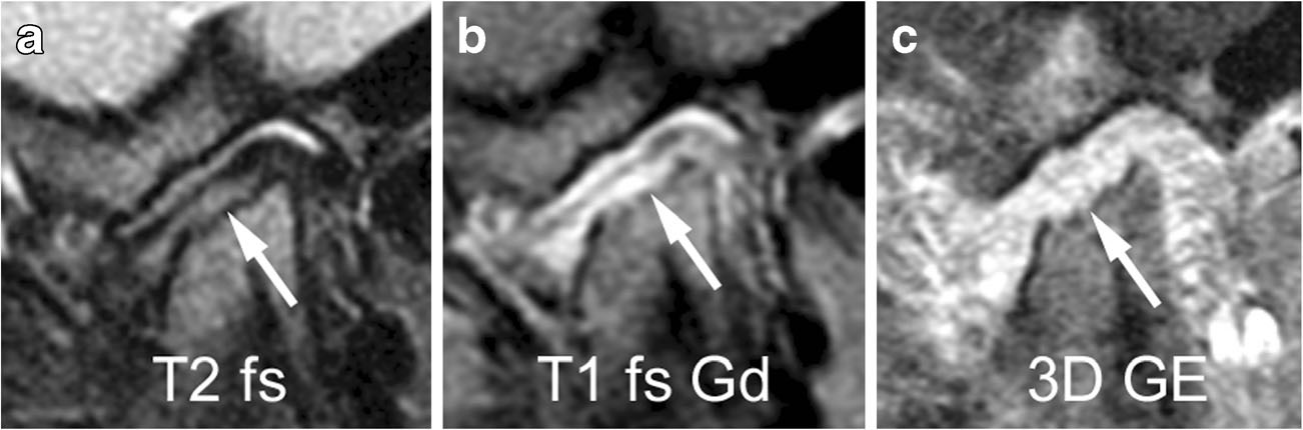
Small erosions in a 7-year-old girl on sagittal oblique images. a-c Irregularity of the bony articular surface (arrows) with small breaks of the subchondral bone is evident on fat saturated fast spin echo image (TR/TE 5,400/77 ms, a) and on fat-saturated postcontrast T1-weighted image (TR/TE 670/10 ms), but is best delineated on gradient echo image (TR/TE 10/4.2 ms, flip angle 20°, c). For assessing the extent of these erosions (part of condylar surface versus entire articular surface), all successive images covering the condyle from lateral to medial need to be viewed. Note bone marrow oedema, mild synovial thickening and severe joint enhancement. 3D GE volumetric T1-weighted gradient echo, T1 fs Gd contrast-enhanced fat saturated T1-weighted, T2 fs fat saturated T2-weighted
